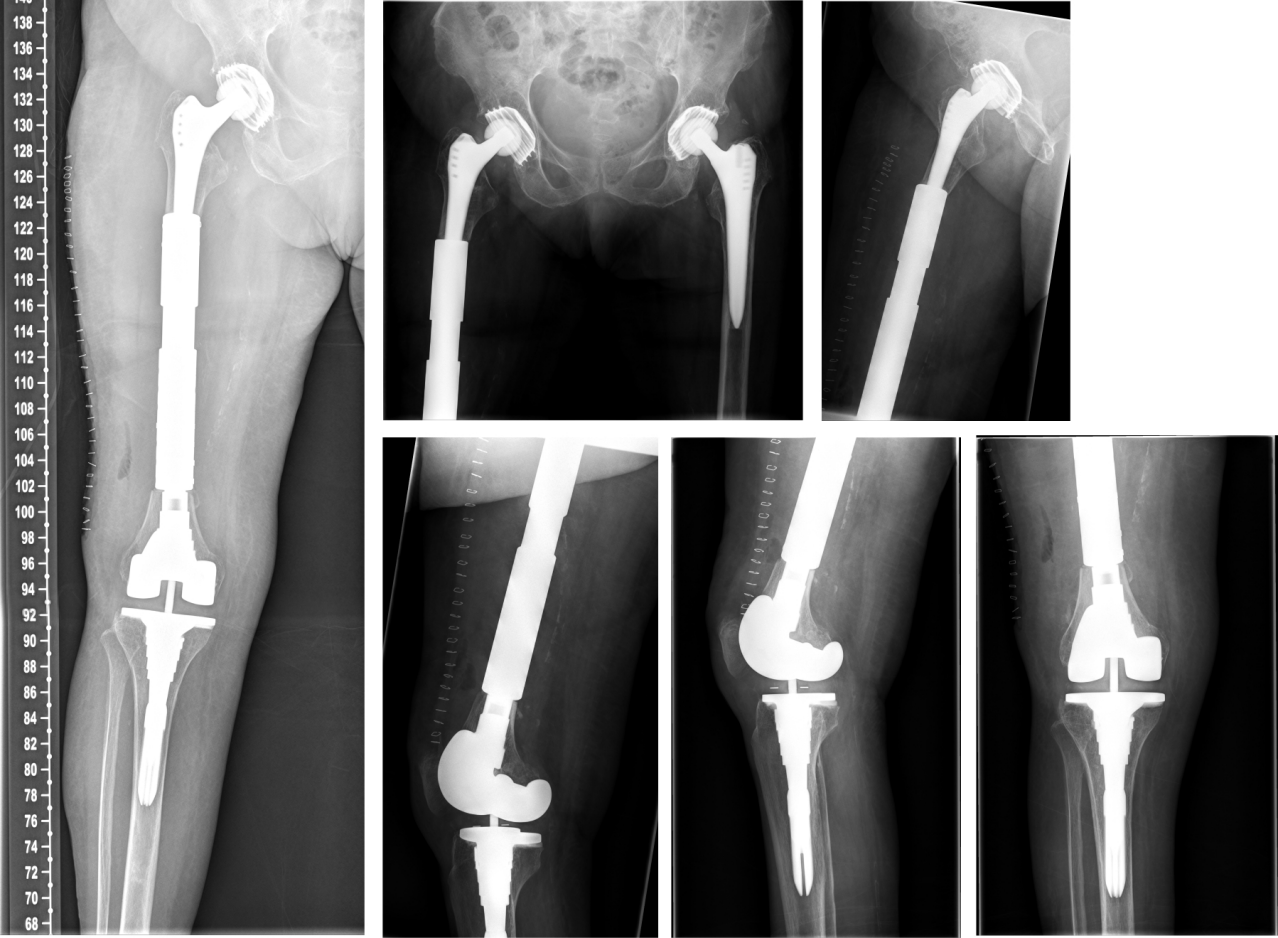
The postoperative radiographs showed correct implant.
